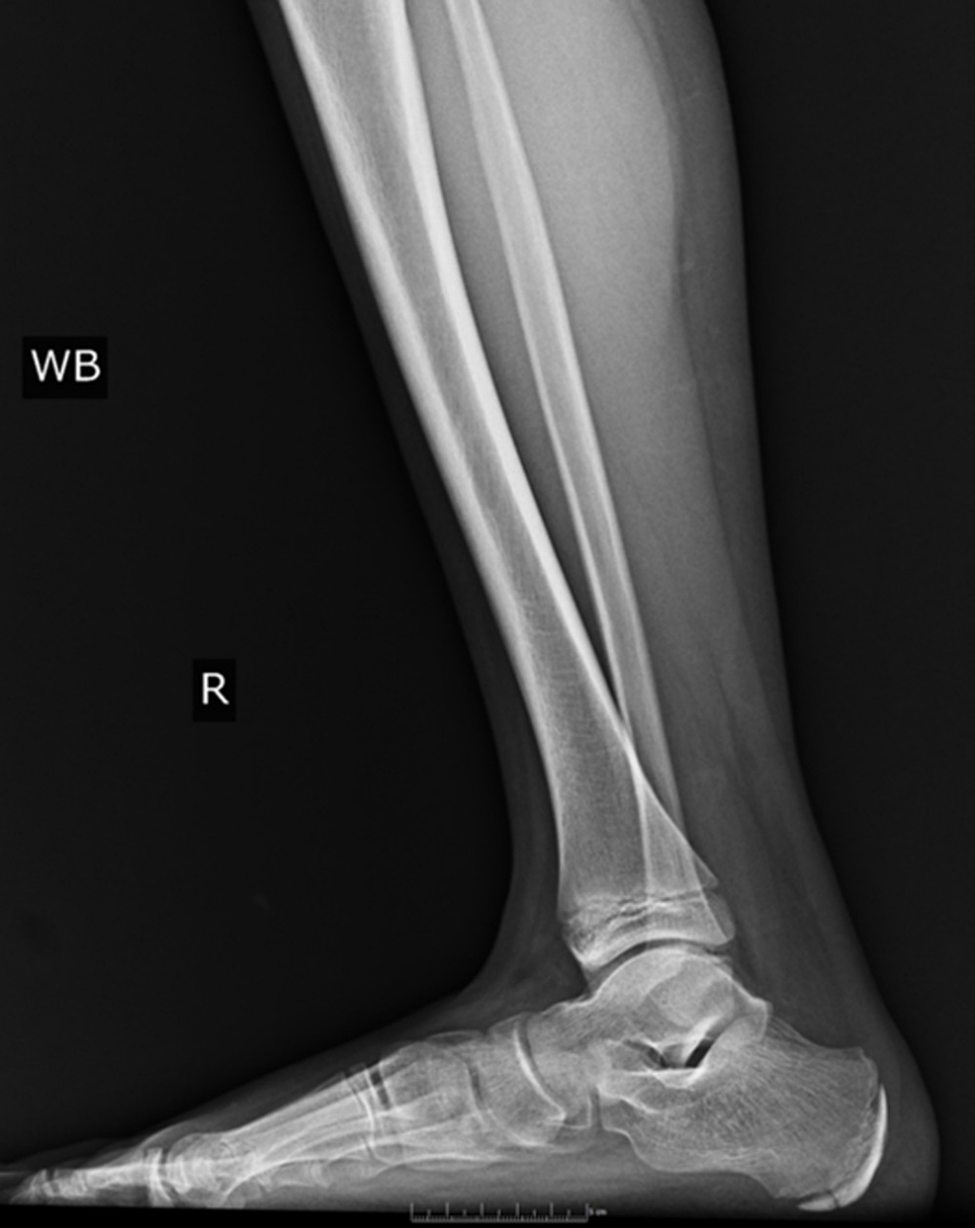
Lateral preoperative right foot weight-bearing X-ray.
